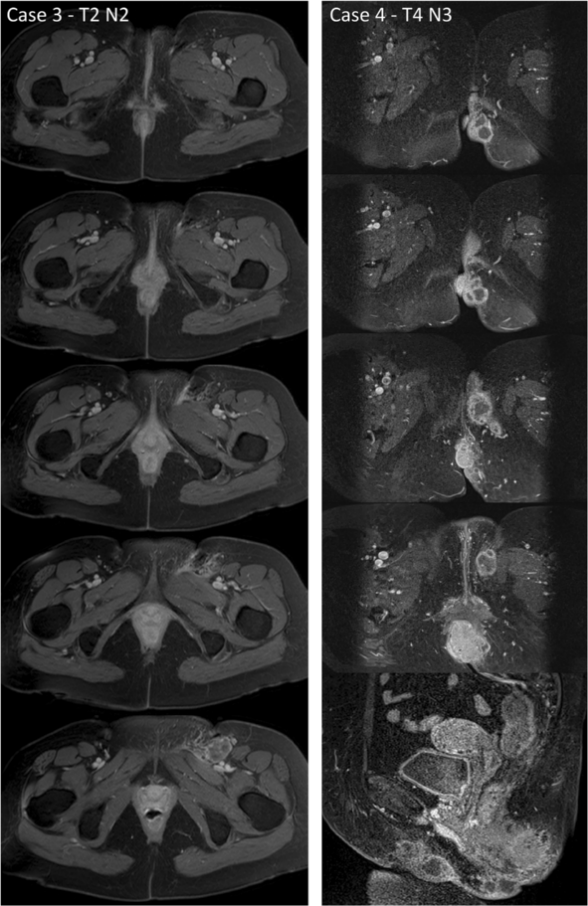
Perigenital involvement in anal carcinoma: MR images Legend: Representative MR images of case 3 (*left*) and case 4 (*right*) in transversal and sagittal planes showing perigenital involvement in anal carcinoma in a T1 weighted sequence after administration of contrast agent
